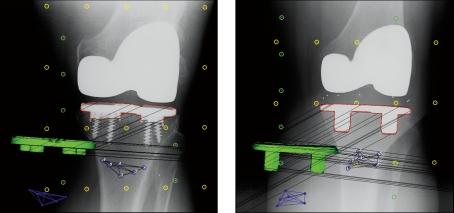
One half of an analyzed model-based RSA image showing the 3-D surface model of the implants (green) with the detected implant contour (red) in the radiographs, and the numbered bone markers (blue) with the corresponding 3-D model (blue). Left: The titanium fiber-mesh screw-fixed tibial component. Right: The double-pegged trabecular metal tibial component.
